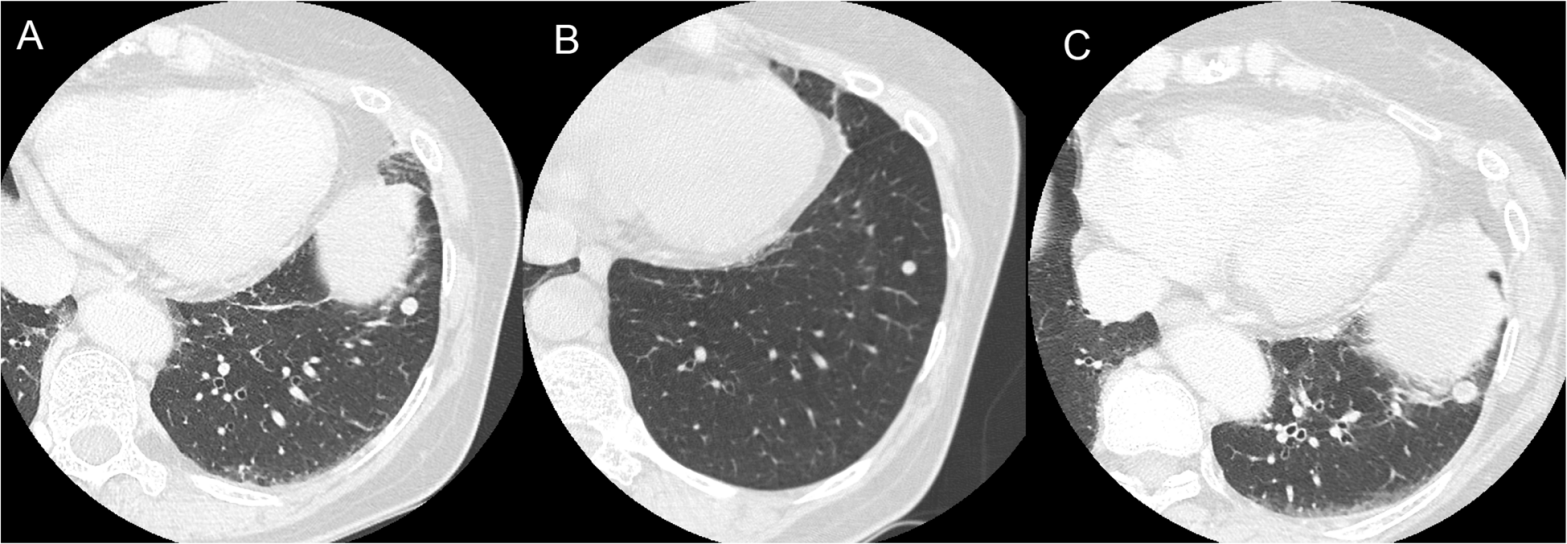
Computed tomography showing a peripheral well-circumscribed pulmonary nodule in the left lower lobe. a The size was 7.0 × 6.6 mm before the thyroidectomy. b It decreased in size to 5.5 × 5.0 mm after the adjuvant radioactive iodine therapy. c It grew to 8.7 × 8.0 mm over 5 years
